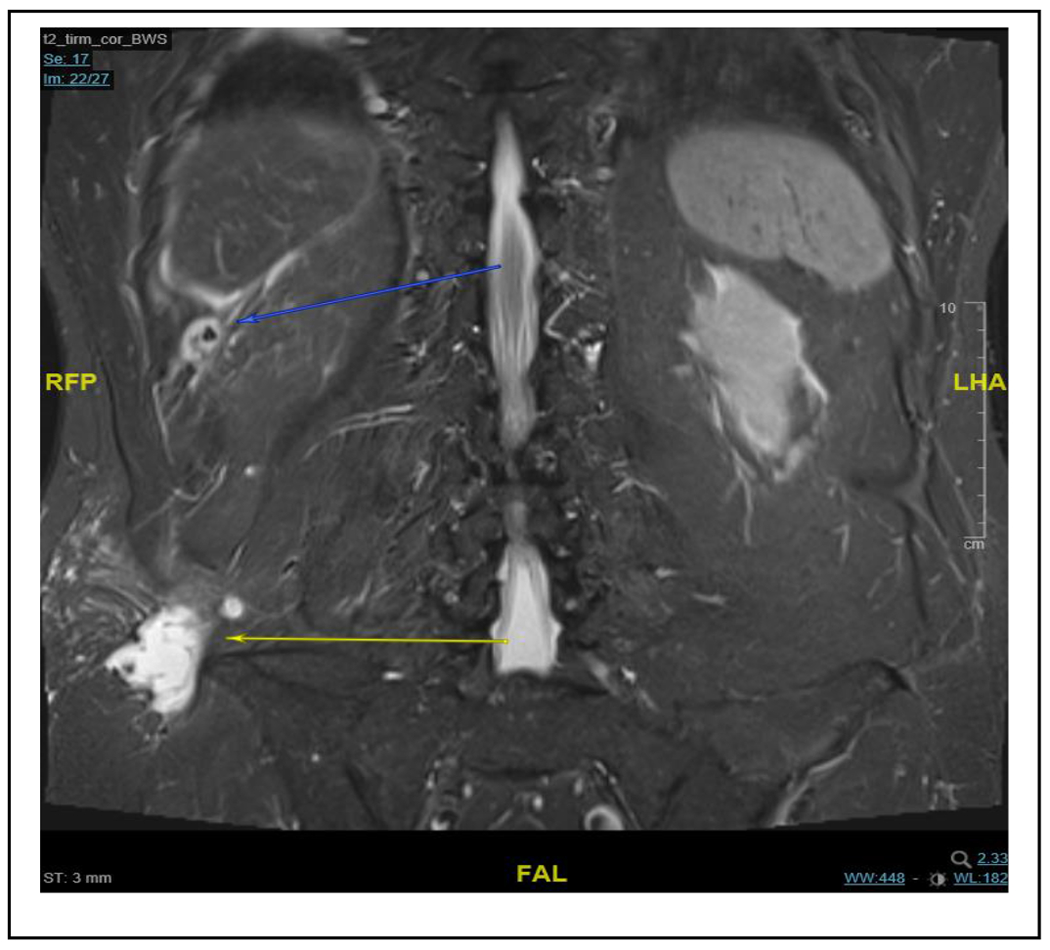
The coronal plane of the MRI scan shows a hyperintense and central hypointense formation caudal to the liver (blue arrow), adjacent to a septated collection alongside the paracolic gutter forming a hyperintense region cranially of the right iliac crest extending dorsally through the lumbar triangle (yellow arrow).
